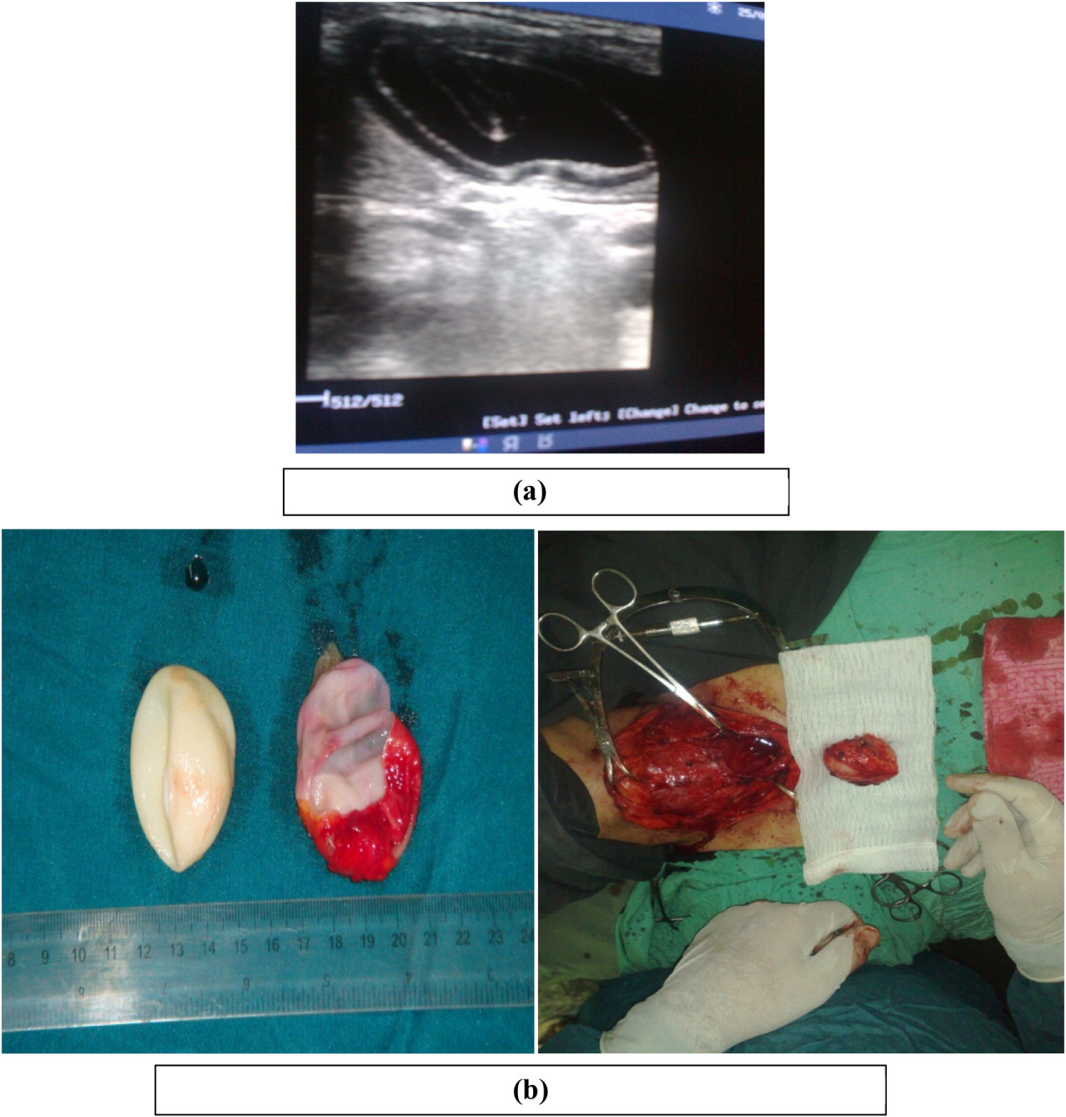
(a) Ultrasound showed a cystic lesion in the anterior neck. (b) Intraoperative picture showing hydatid cyst in the anterior aspect of the neck. The photos are taken from patient number 7.
